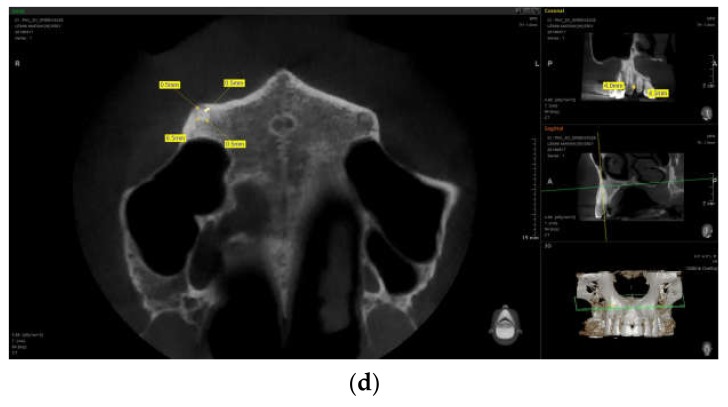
Measurements of periodontal ligament space on cone beam computed tomography (CBCT): (a) clinical attachment level (CAL) and apical PDL space measurements; (b) 8-point cervical third measurements; (c) 4-point middle third measurements; and (d) 4-point apical third measurements.
